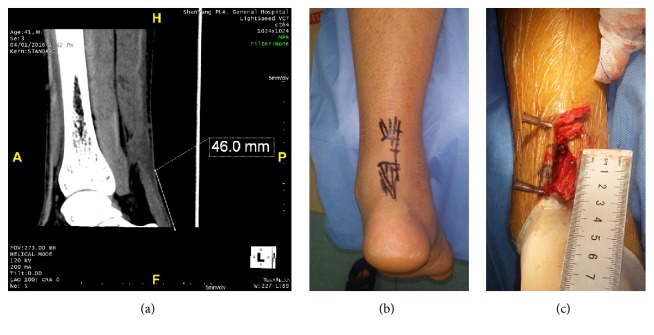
41-year-old male. (a) Adjusting the axis of images though the MPR module and measuring the length of distal part. (b) Locating the rupture position by the distance from the calcaneal insertion of AT measured in CT MPR images. (c) Measuring the length of distal part intraoperation directly.
